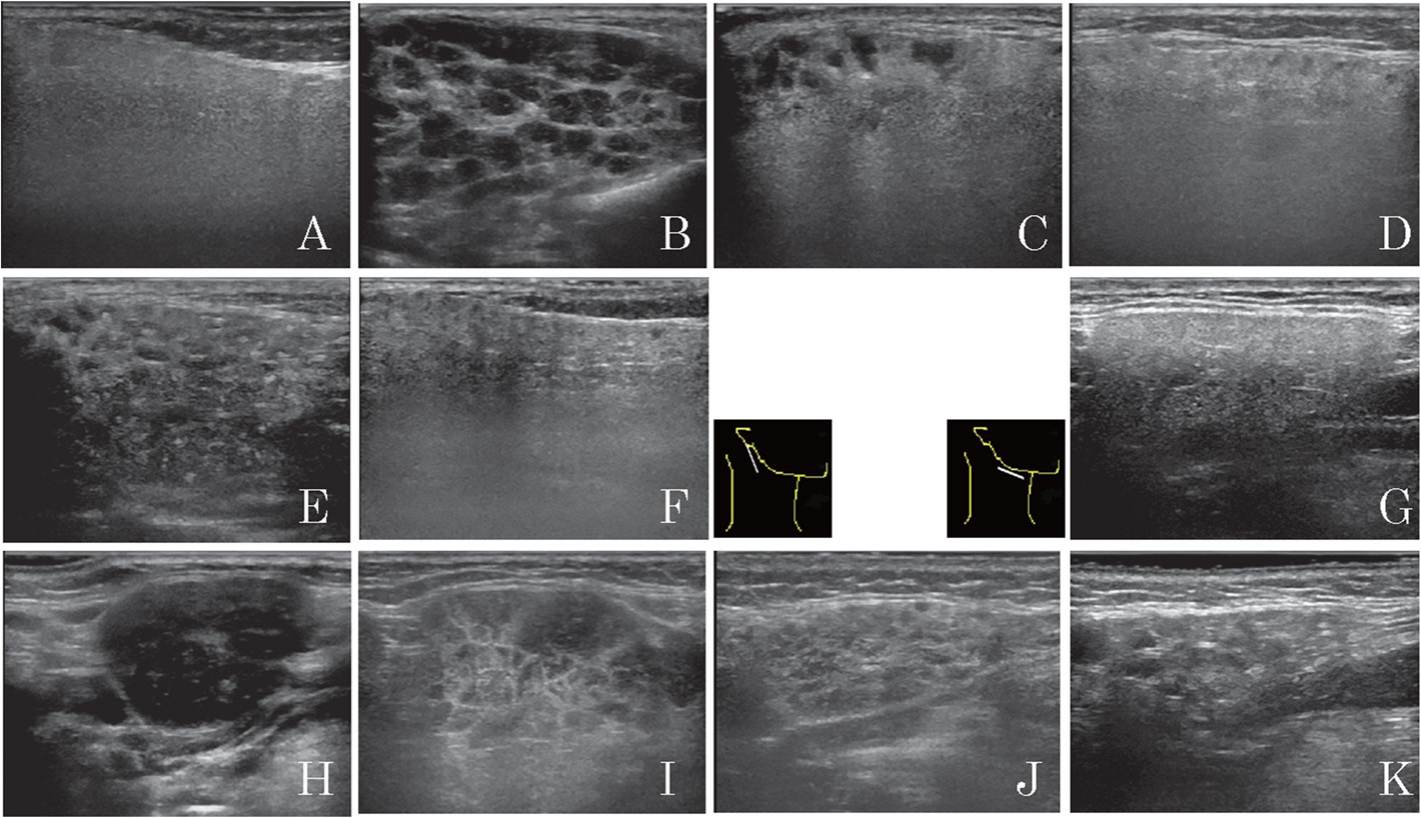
Characteristic findings on sonograms. a Normal parotid gland. b-d Parotid glands of a patient with IgG4-DS. e, f Parotid gland of a patient with SS. g Normal submandibular gland. h, i Submandibular glands of a patient with IgG4-DS. j, k Submandibular glands of a patient with SS. Multiple hypoechoic areas (b-f, i-k), a reticular pattern (b, e, i), and a nodal pattern (h) can be seen. Hyperechoic lines and/or spots can also observed (b-f, i-k). *IgG4-DS* IgG4-related dacryoadenitis and sialadenitis, *SS* Sjögren’s syndrome
